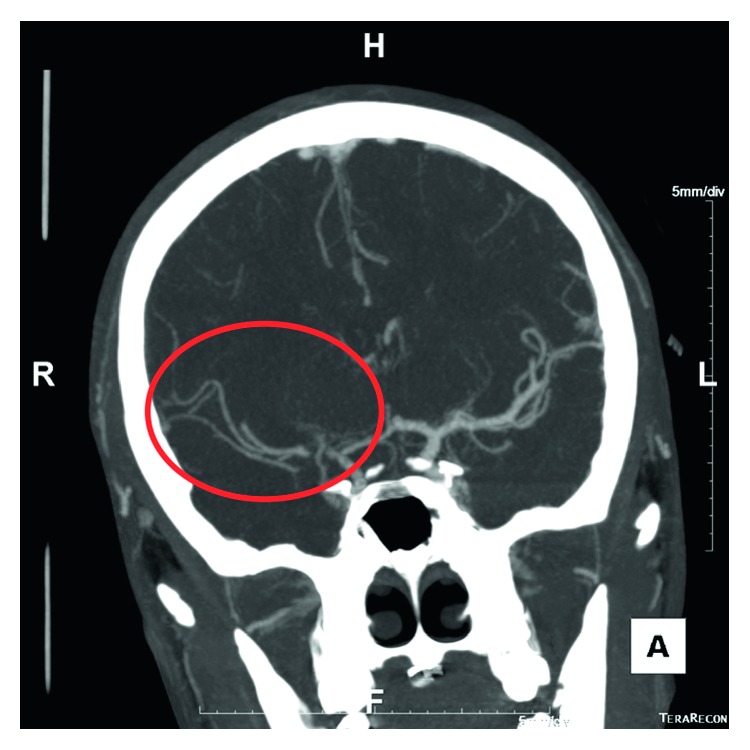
Postthrombectomy, CTA maximum intensity projection (MIP) shows improved blood flow and distal filling of the right MCA.
